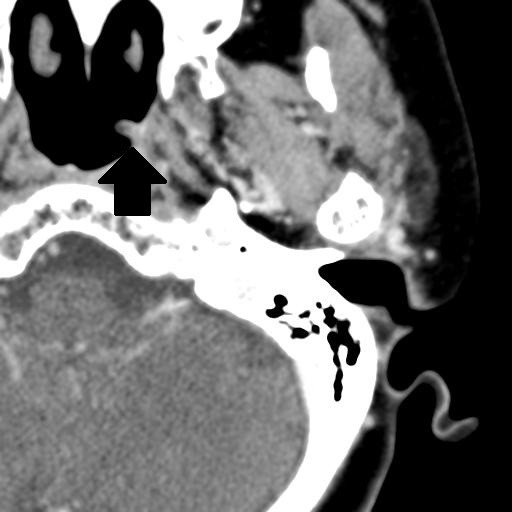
Axial CT images, revealing a small mass almost 5 mm in diameter at the left wall of the nasopharynx.
